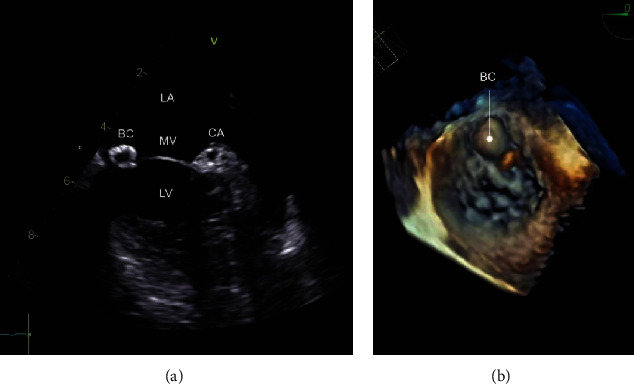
Transesophageal echocardiogram (a) with 3D reconstruction (b), exhibiting a blood cyst attached to the anterior leaflet of the mitral valve. LA: left atrium; LV: left ventricle; MV: mitral valve; BC: blood cyst; CA: circumflex artery.
